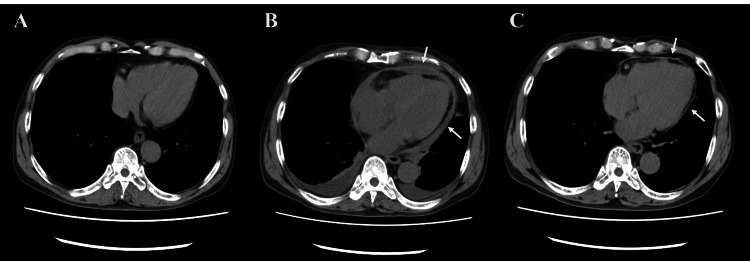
Sequential changes in chest computed tomography. (A) Two years before admission (control). (B) Day 18. Three-layered pericardium (arrow). (C) Day 119. Improved pericardial thickening (arrow).
